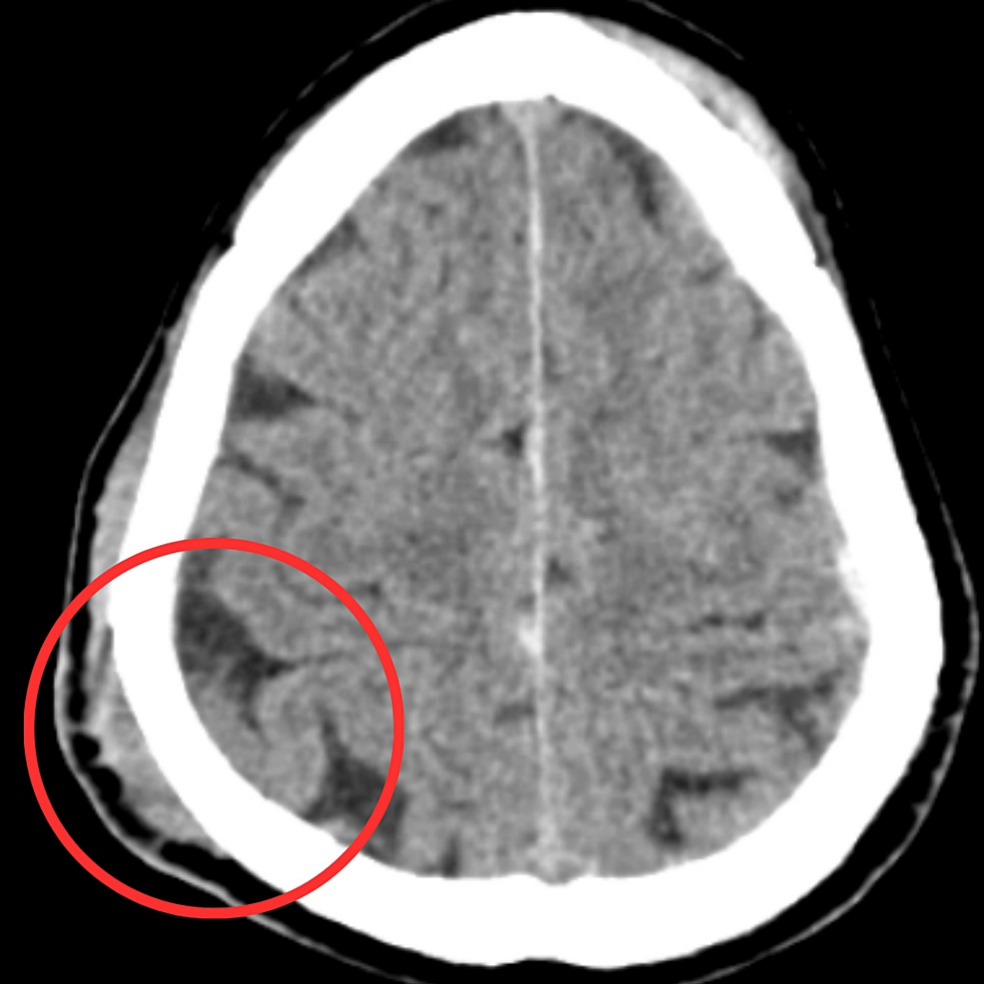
Computed tomography scan of the brain Red circle shows subdural hematoma and subarachnoid hematoma
